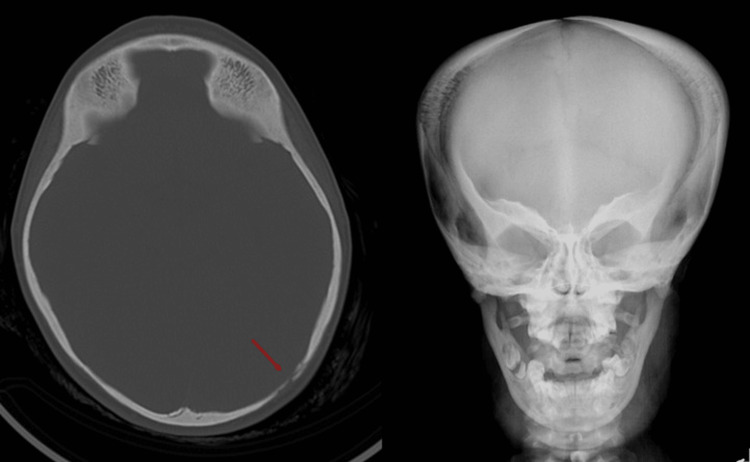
Lower axial CT slice demonstrating heterogeneous bone density and irregular sclerotic margins, with corresponding coronal radiographic view. Arrow marks the deformity margins.
